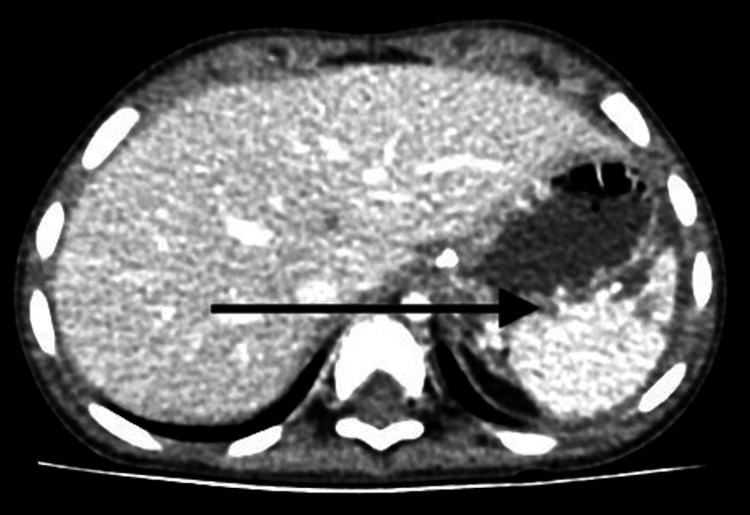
Contrast-enhanced CT demonstrating irregular, dilated, and tortuous vessels extending to the pancreatic tail (arrow).
